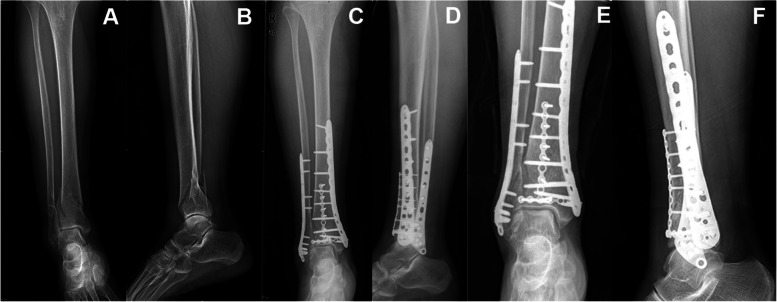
A 41-year-old male with pilon fracture of his left leg from a height fall, Rüedi and Allgöwer II type. A, B Preoperative anteroposterior and lateral radiographs. C, D Anteroposterior and lateral view of postoperative X-ray. E, F Anteroposterior and lateral X-ray 15 months after surgery
